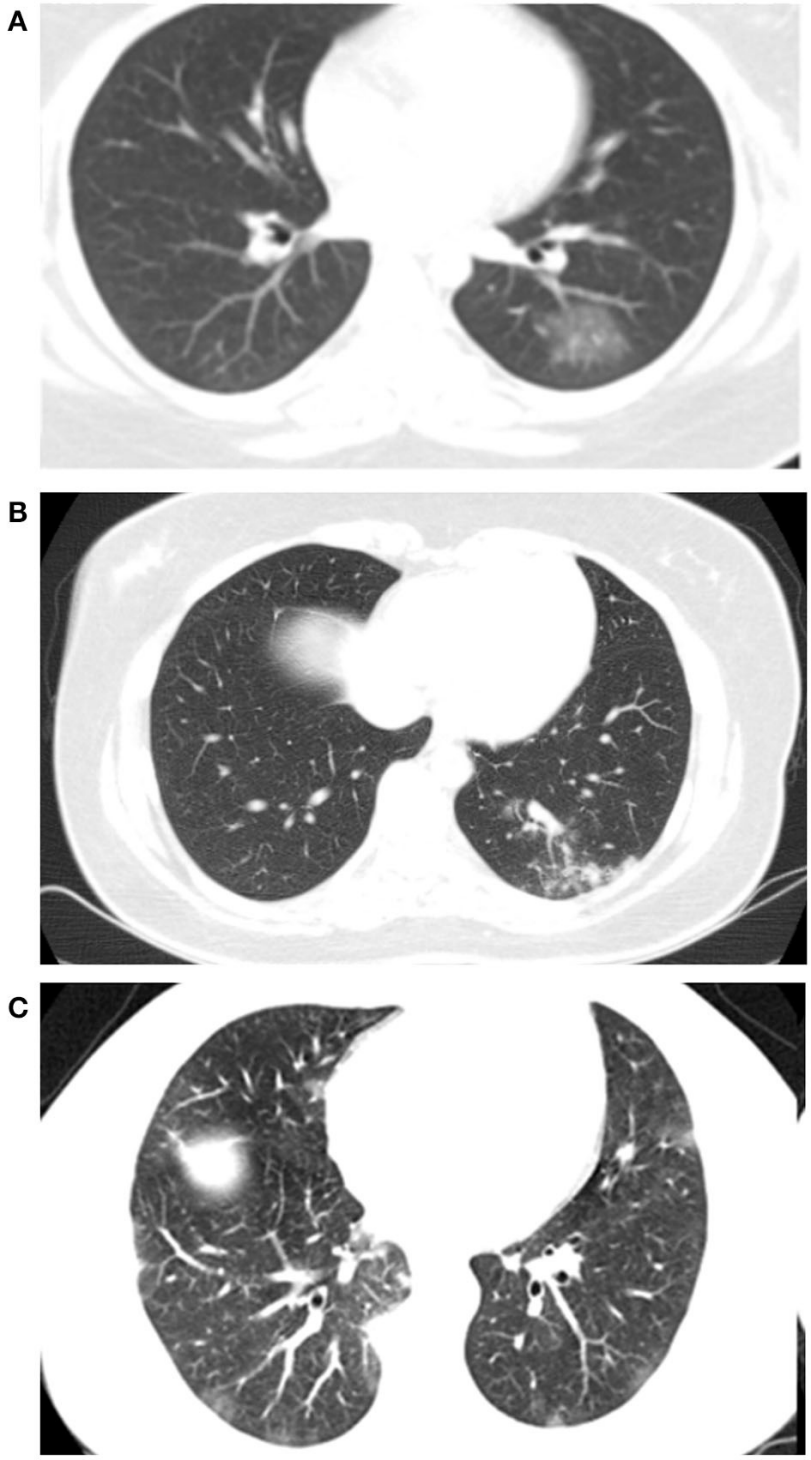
Patients chest CT showing coronavirus disease 2019 reporting and data system (A) CORADS 3. (B) CORADS 4. (C) CORADS 5.
